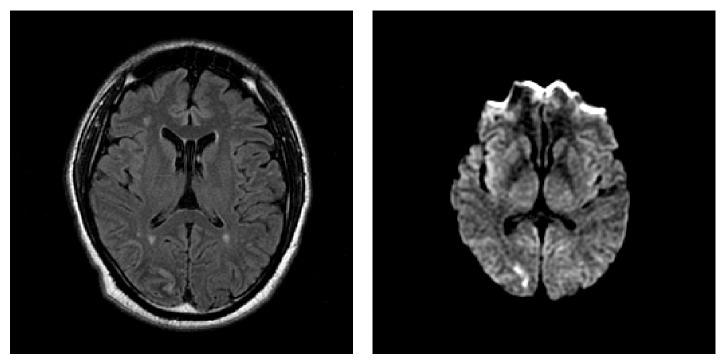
MRI 2 days after onset of symptoms, presenting acute occipitotemporal ischemic injury, corresponding to PCA territory sparing Brodmann area 17, and involving ventral portion of occipitotemporal projection; Brodmann areas 18 (parastriate) and 19 (peristriate).
